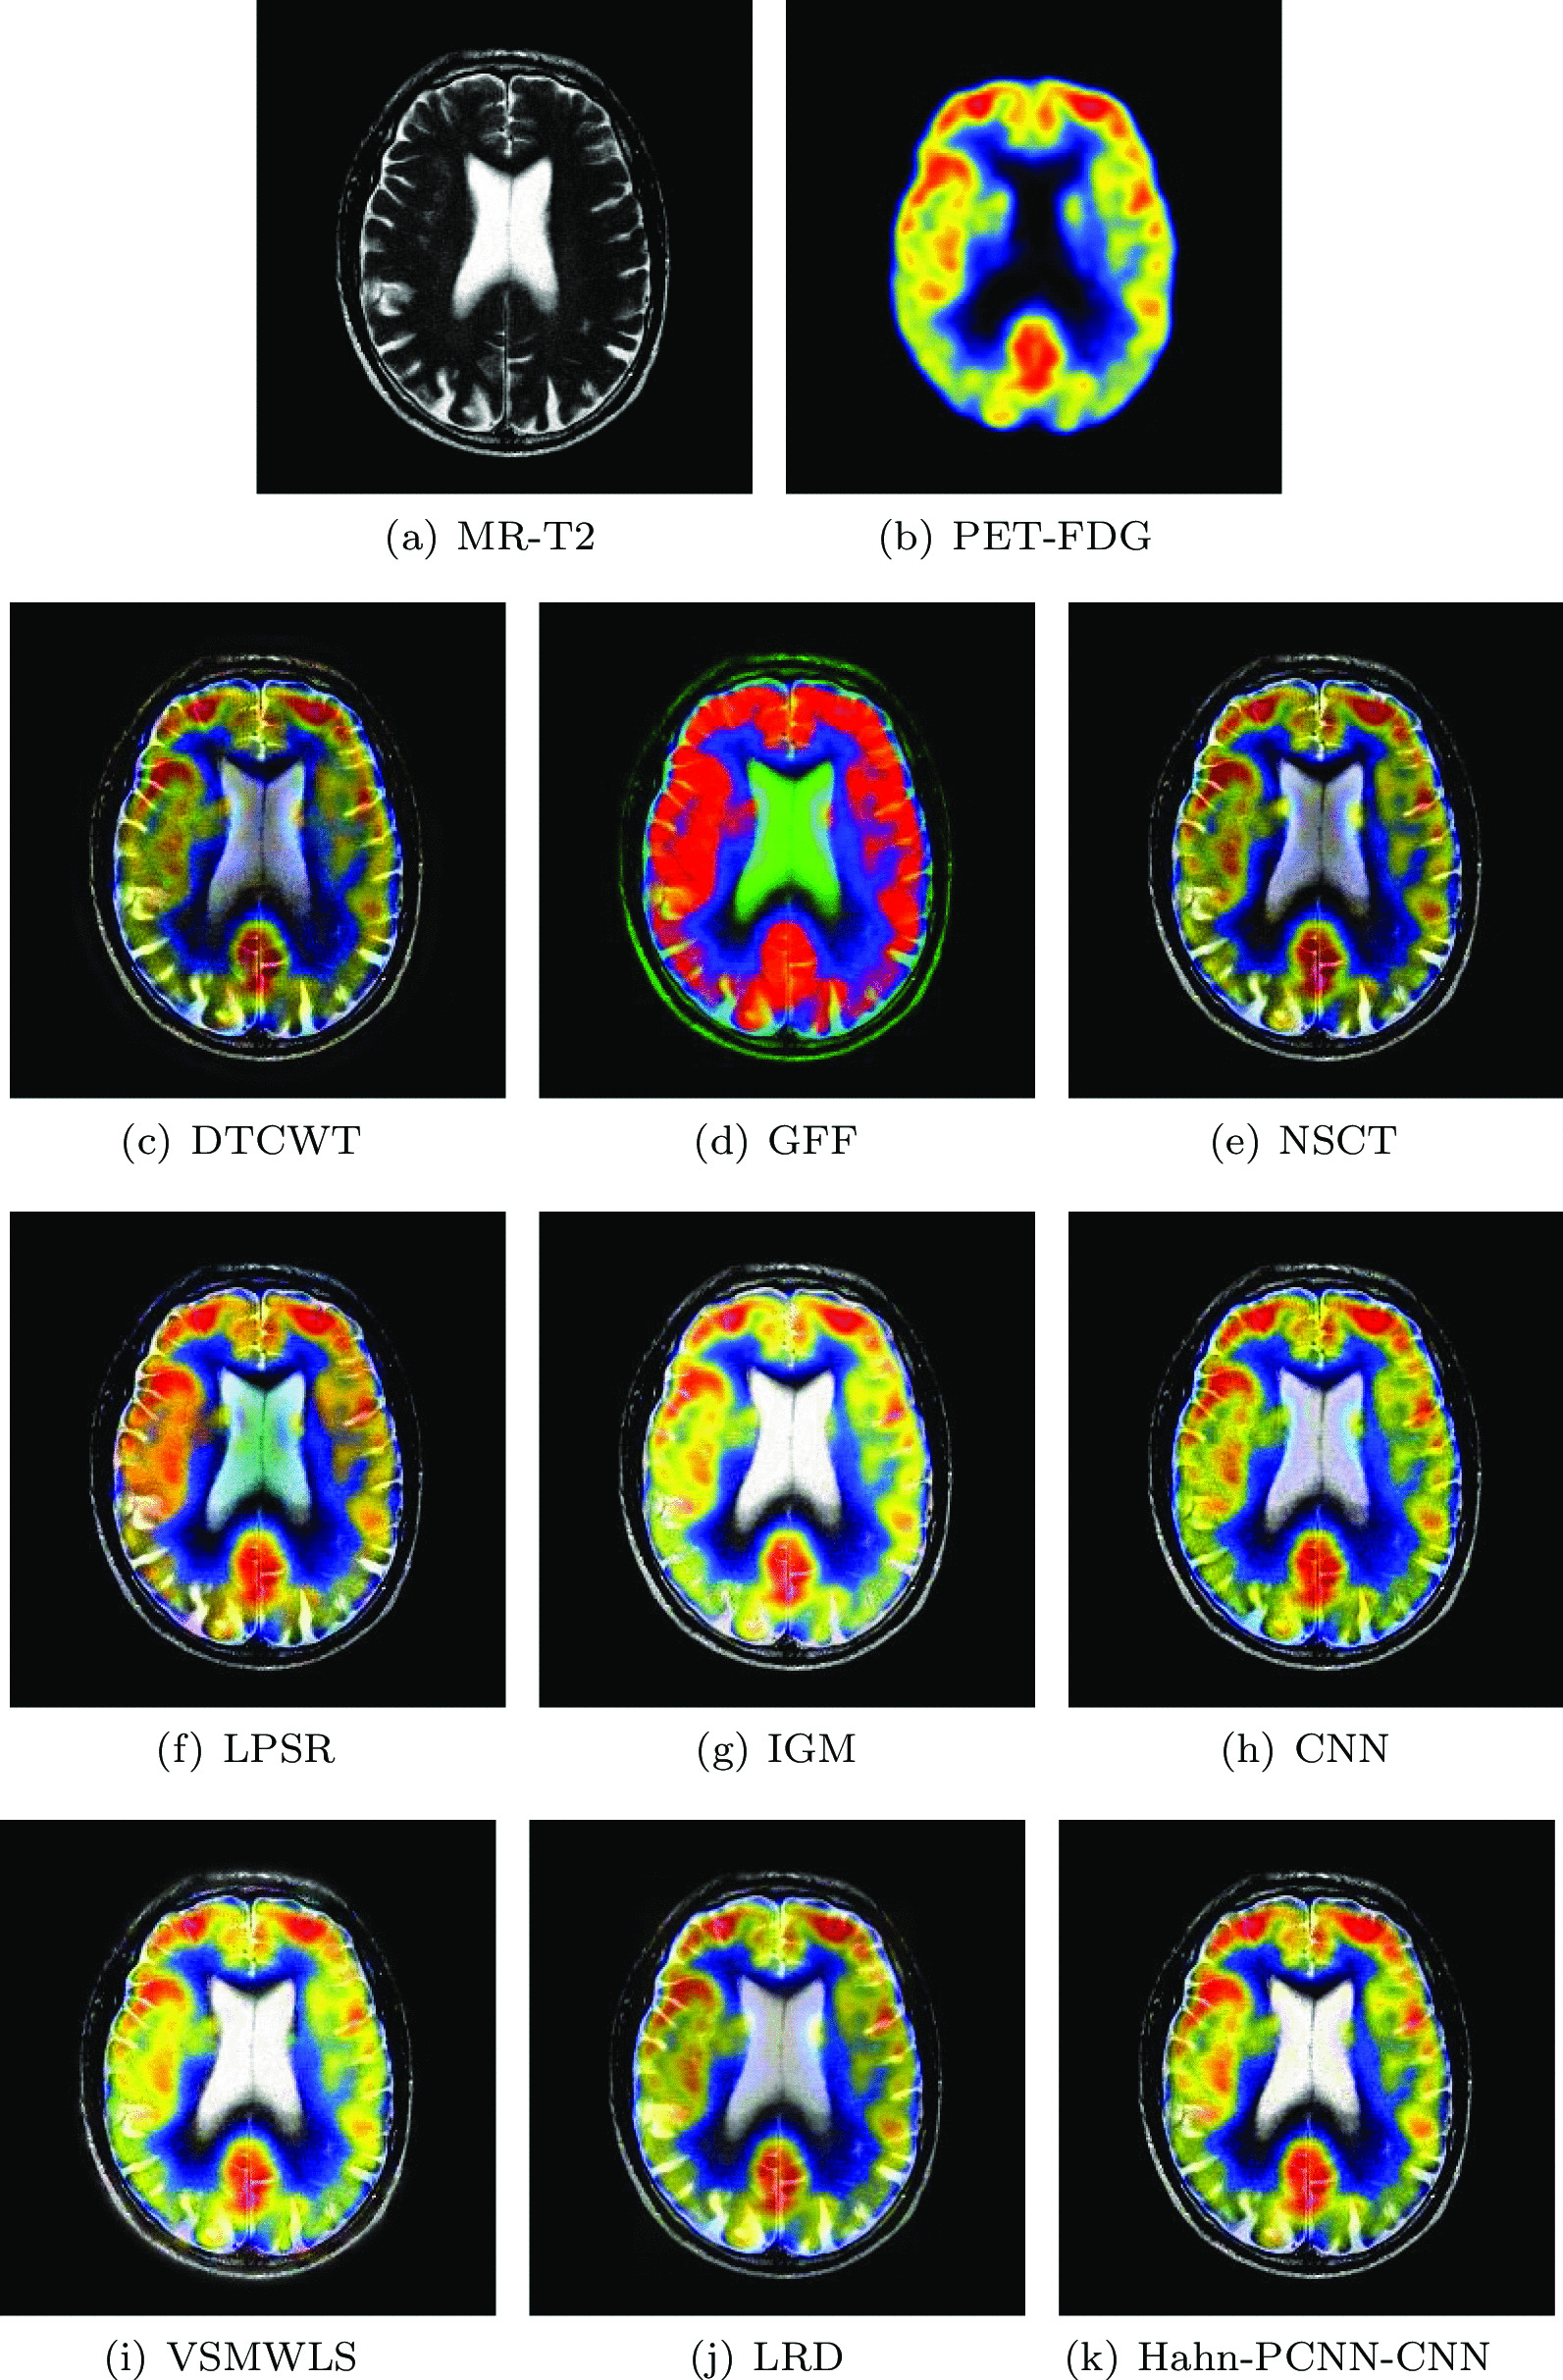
The first set of fused MRI-PET images from 9 methods on mild Alzheimer’s disease
